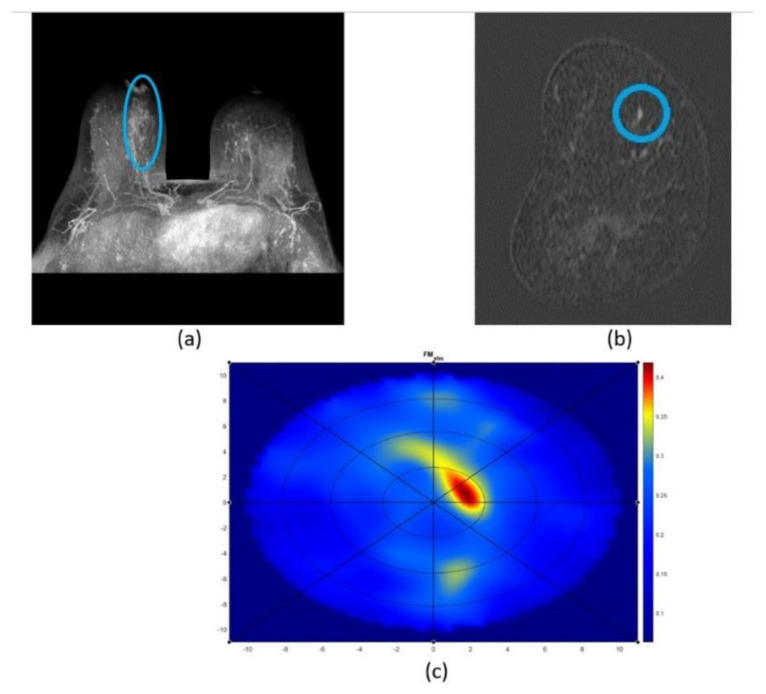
Imaging performance of MRI and SAFE: (a,b) MRI axial and coronal planes showing the presence of malignant lesion in non-dense breast, and (c) SAFE coronal plane confirming the presence of malignant lesion (high contrast region), inside the scanning domain (blue circle), at the same location as reported by MRI.
